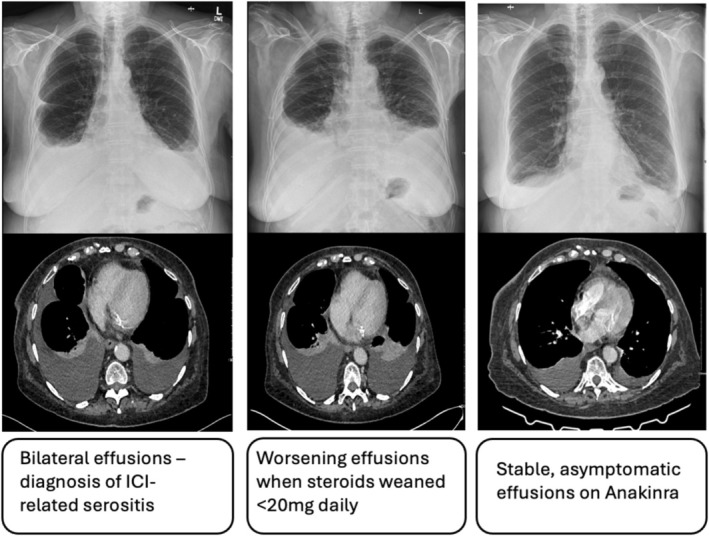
Serial chest radiographs and axial CT images show bilateral pleural effusions from immune checkpoint inhibitor–related serositis, worsening with corticosteroid taper and stabilizing after initiation of anakinra.
